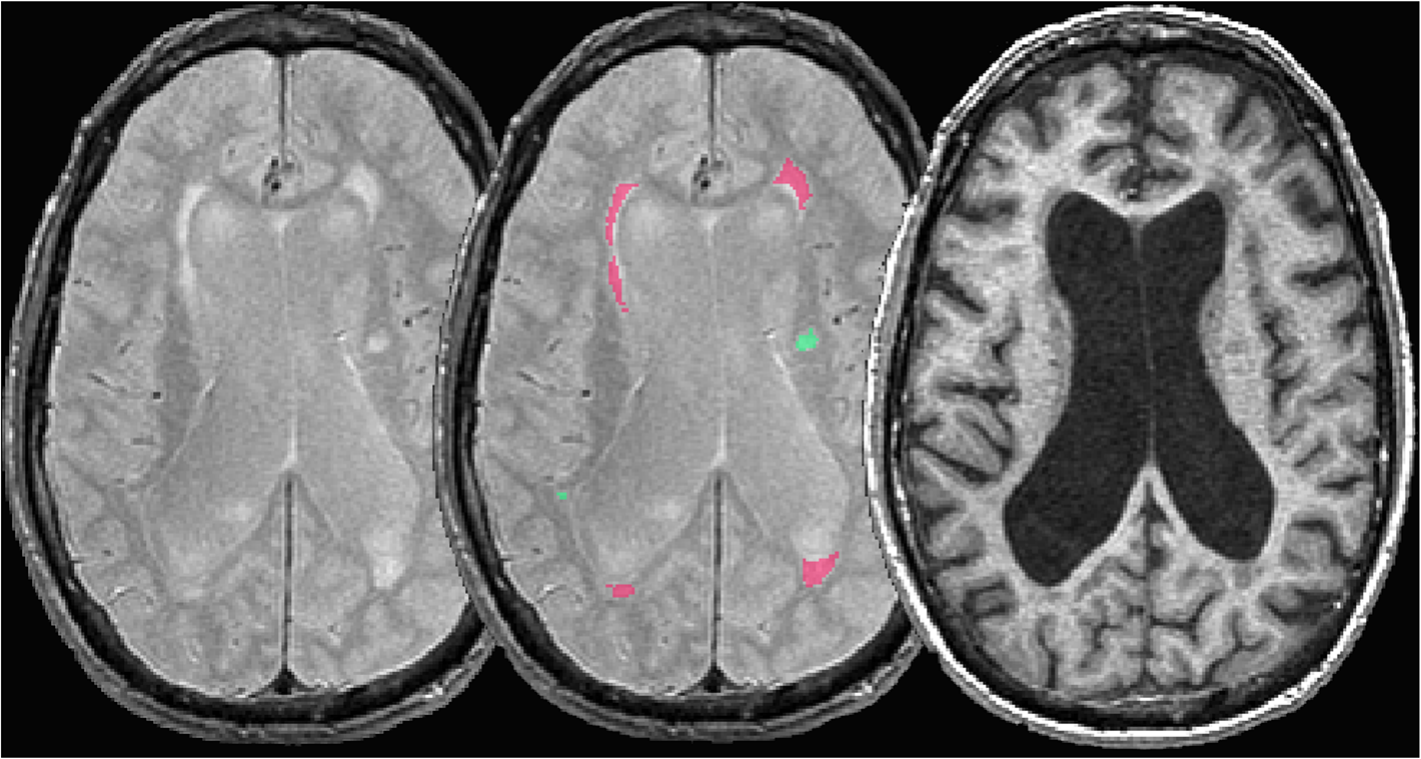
Segmentation of periventricular subcortical hyperintensity (pink) and deep white subcortical hyperintensity (green) overlayed on coregistered proton density (center) and T1-weighted (right) magnetic resonance imaging.
